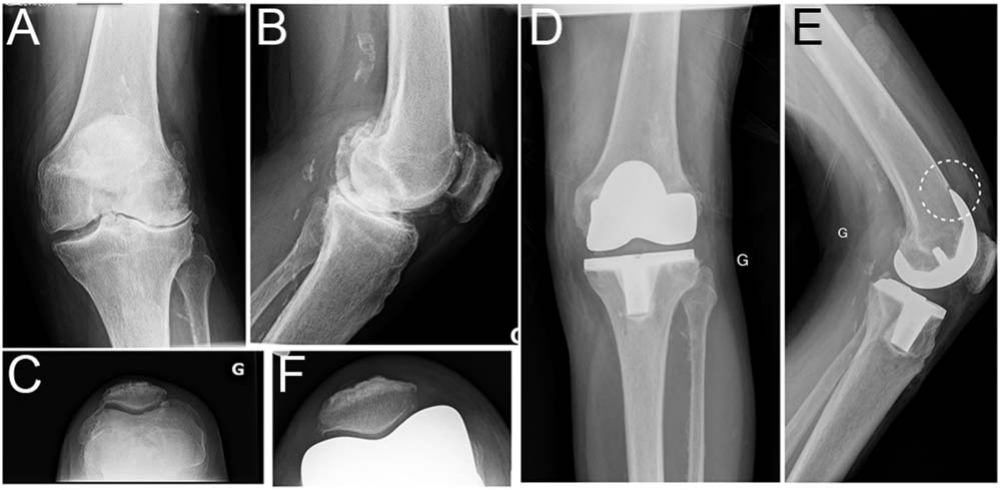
Trochlea dysplasia. (A, B, C) Preoperative long-leg radiographs of an 86-year-old male with a moderate valgus left knee. The knee was presenting a shallow and dysplastic trochlea. (D, E, F) During surgery, femoral size was between size 6 and 7. We selected the smaller size with a posterior reference and voluntarily accepted to notch the anterior cortex by 2 mm to avoid overstuffing the dysplastic patellofemoral joint (dashed circle). Following the rKA protocol, we resurfaced the medial femoral condyle (implant thickness from zones of intact cartilage) and removed 7 mm from the subchondral bone on the lateral compartment to reduce the femoral valgus from 6 to 5°. Tibial cut angulation was 3° for an arithmetic HKA of 2° valgus. The knee was stable, and a medially constrained CR insert was selected.
